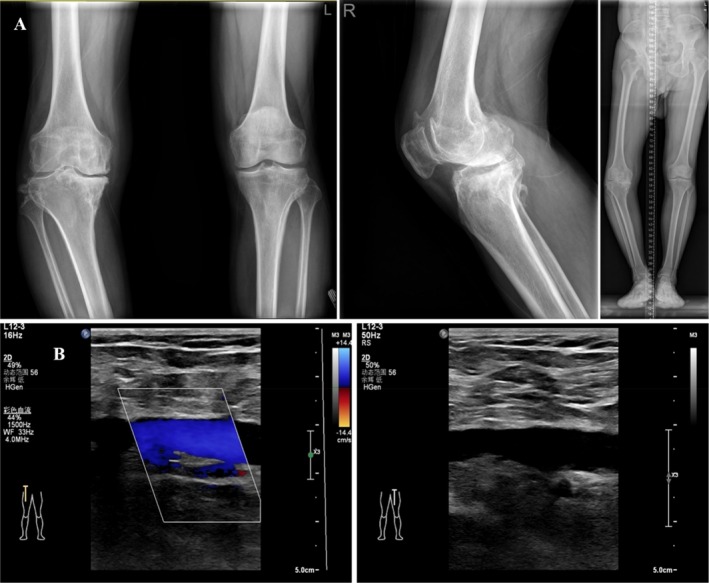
(A) Pre‐operative X‐rays of the right knee. X‐rays revealed right tri‐compartmental advanced osteoarthritic changes in the right knee, including medial joint space narrowing, subchondral sclerosis, and osteophyte formation. (B) Pre‐operative double lower limb deep vein ultrasound. The inner diameter of the deep veins in both lower limbs was normal, the endothelium was smooth, and there were no obvious abnormal echoes in the venous lumen.
